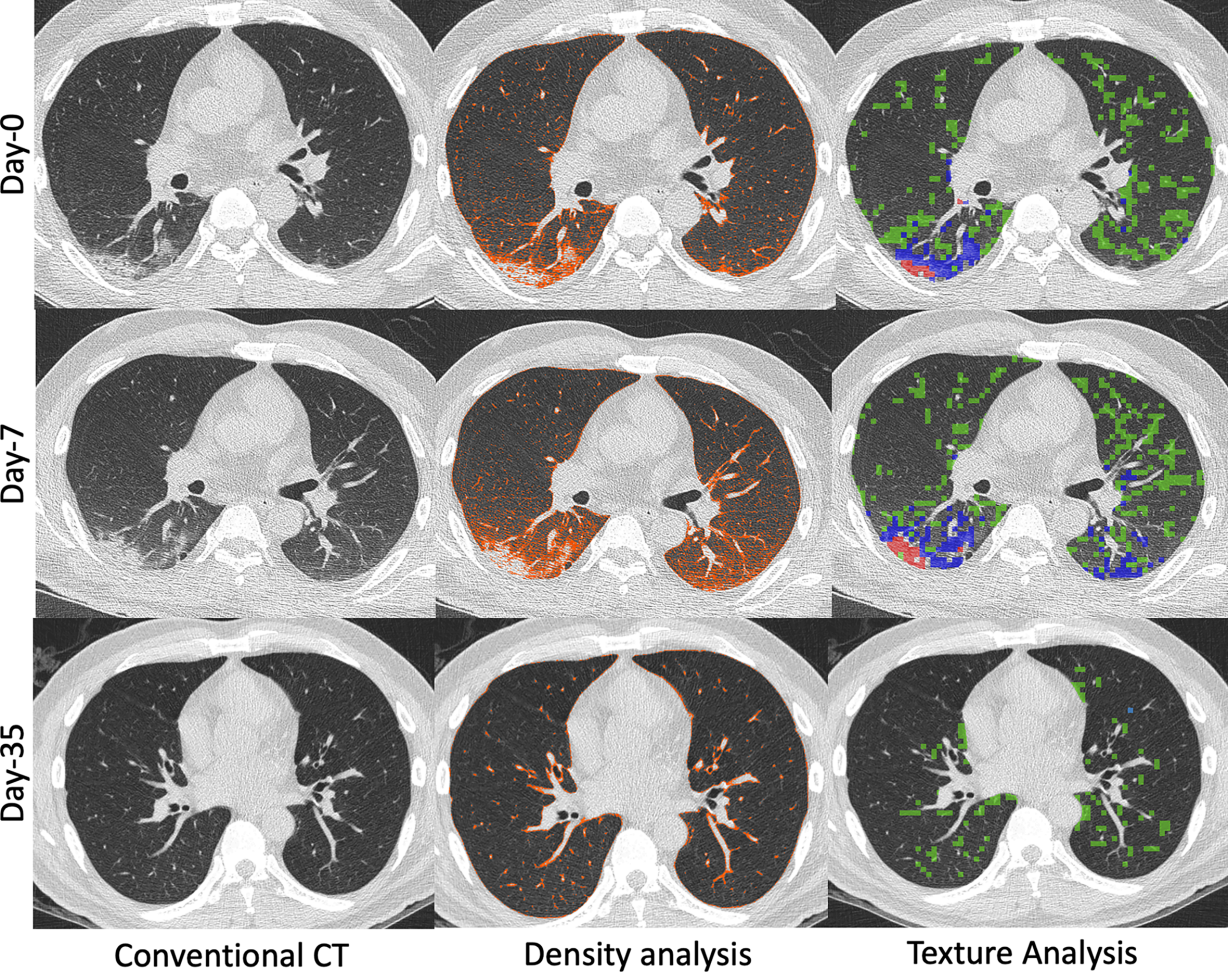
55-year-old male with COVID-19 pneumonia that received imaging at presentation (Day 0), Day 7 of hospitalization, and Day 35 of hospitalization. Images generated at initial presentation (Top row, Day 0) along with computed density and texture analysis demonstrating predominantly posterior bilateral lower lobe disease (HAAs quantified as 13% on density analysis and abnormal lung quantified as 24% on texture analysis). The texture analysis characterized the disease as GGO (green, 21%), mixed GGO and interstitial (blue, 3.9%), and consolidation (red, 0.1%). Follow-up CT (Middle row, Day 7) obtained due to worsening shortness of breath demonstrated minimal disease progression on conventional CT images and density analysis and texture analysis provided objective assessments of disease worsening (HAA on density analysis quantified as 18% and abnormal lung quantified as 29% on texture analysis). Progression of imaging disease type was also quantified (GGO, green: 22.5%; mixed GGO and interstitial, blue: 6.3%; and consolidation, red: 0.2%). Another follow-up CT (Bottom row, Day 35) obtained after clinical improvement and prior to discharge from the hospital demonstrated complete resolution of disease on conventional images. The density and texture analysis identified some residual abnormalities (HAA 5% on density analysis and abnormal lung 6% on texture analysis). GGO, ground glassopacity; HAA, high attenuation area
